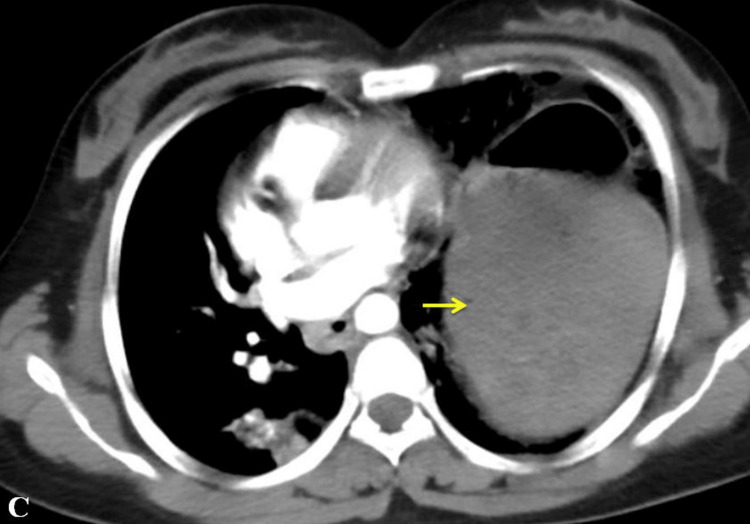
CT thorax showing herniated stomach in the left hemithorax (arrow) air-fluid level.
